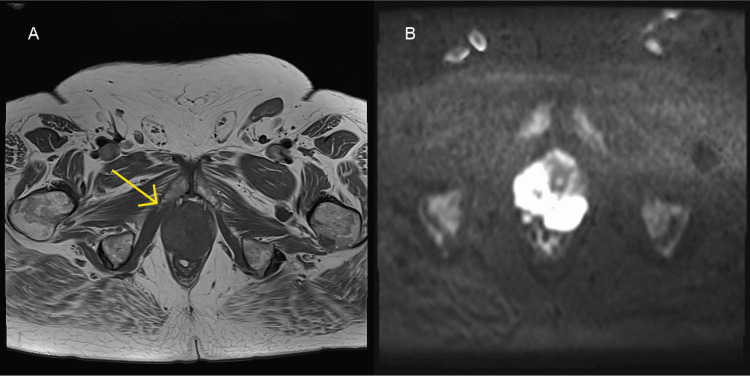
Multiparametric MRI of the prostate. (A) Axial T2-weighted slice demonstrating a focal hypointense lesion at the apex of the prostate (yellow arrow); (B) Diffusion-weighted image demonstrating hyperintensity at the same location consistent with restricted diffusion.
